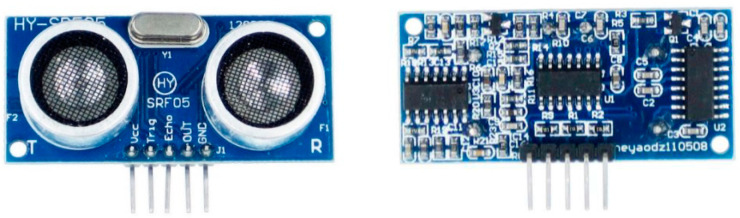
Low-cost ultrasonic distance sensor (HY-SRF05). R and T indicate the receiver and transmitter, respectively.
